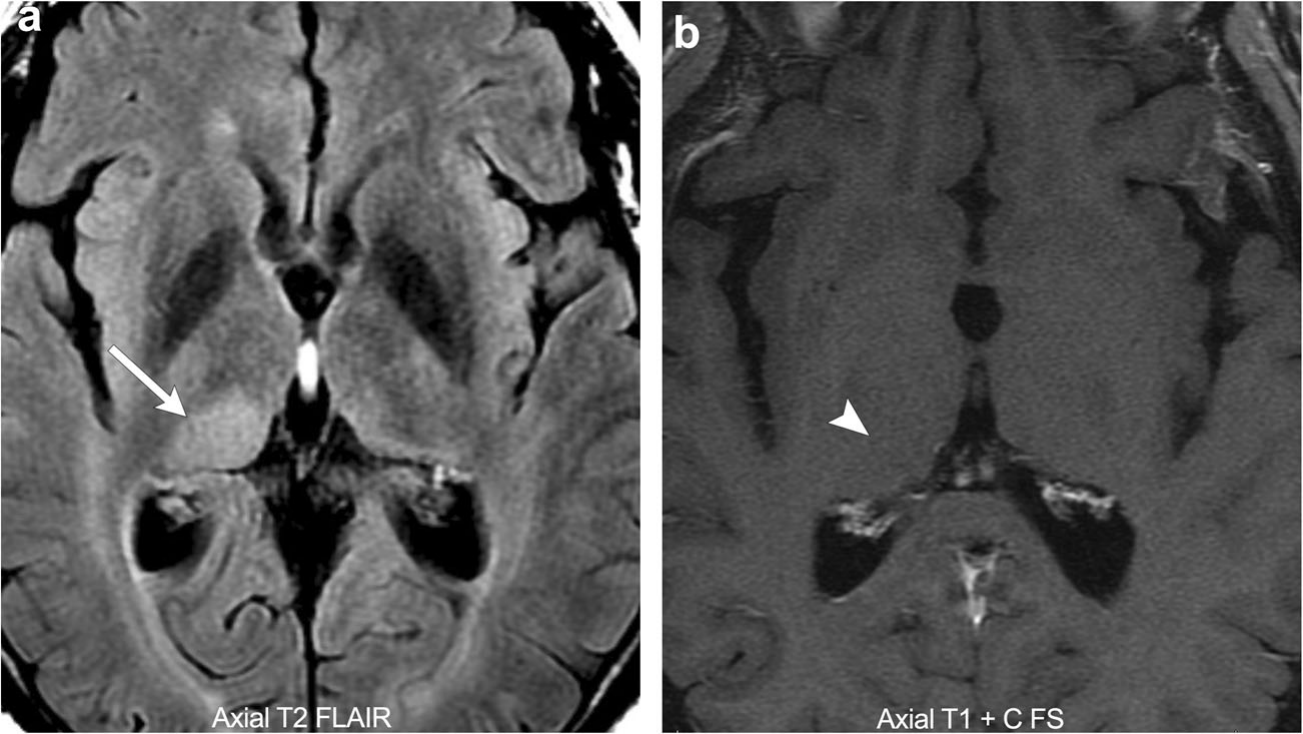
Pathology-proven low-grade glioma. A 58-year-old male with a T2 FLAIR hyperintense mass in the right thalamus (arrow). No associated enhancement (arrowhead)
